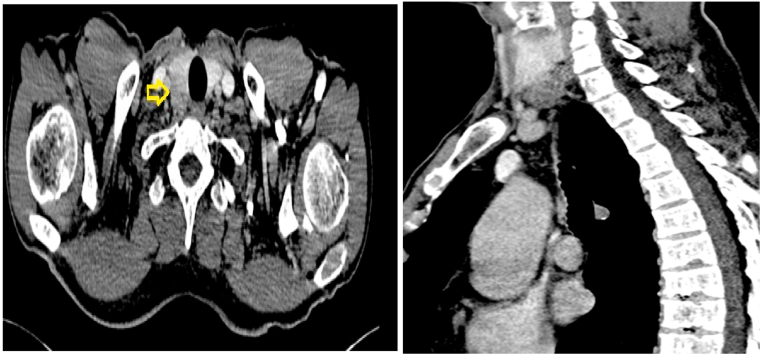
(A and B) Cervical computed tomography scan showing a hypodense retro-thyroid mass (arrow). A: axial section. B: sagittal section.
